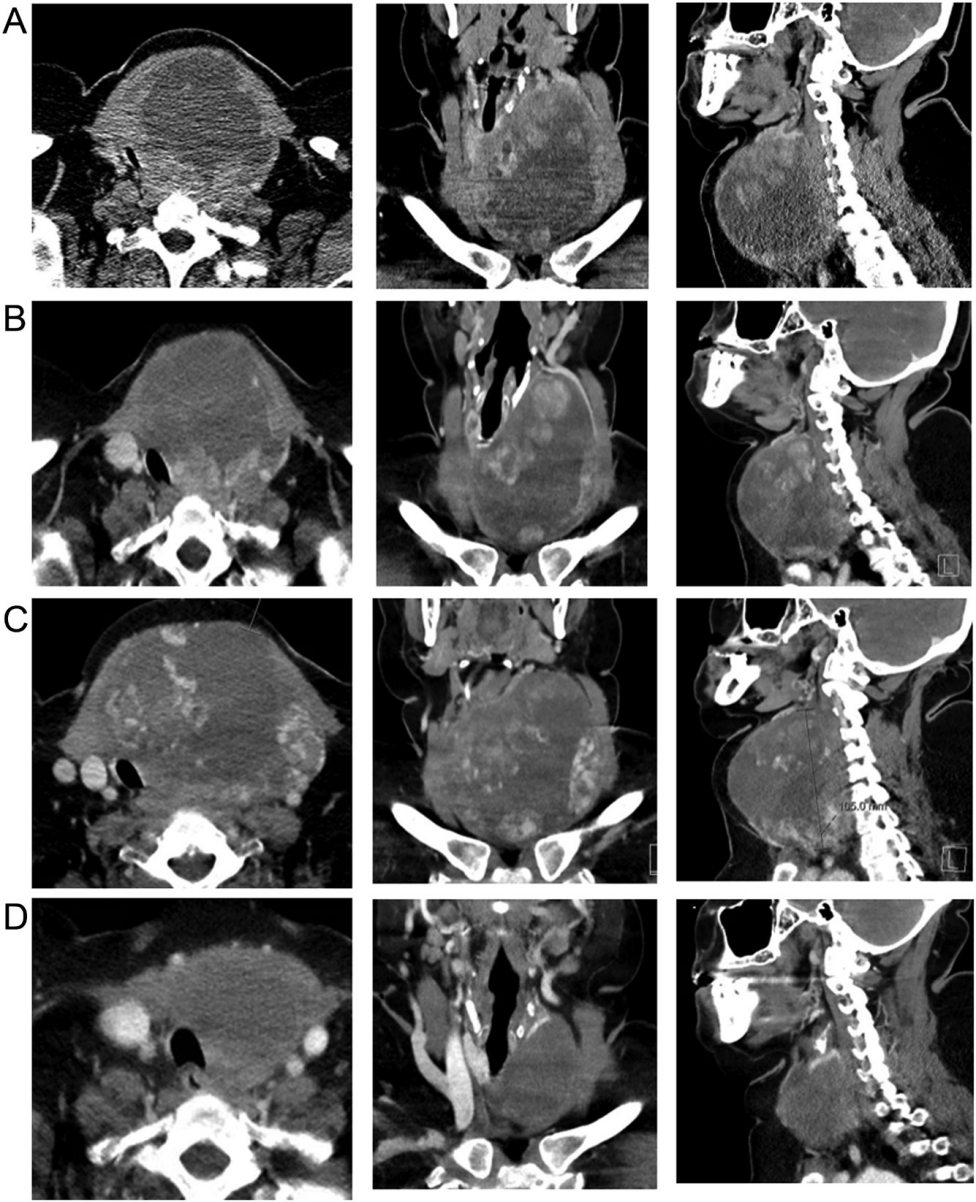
Neck CT scans showing the effect of lenvatinib and entrectinib on tumor size. (A) Before lenvatinib – May 2021. (B) After 2 weeks of lenvatinib – June 2021. (C) After 12 weeks of lenvatinib – August 2021. (D) After 9 weeks of entrectinib – October 2021.
